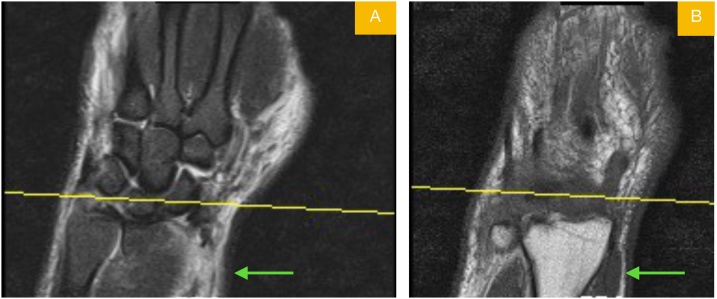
First dorsal compartment retraction coronal view of MRI T1 (A) and T2 (B) sequences.
